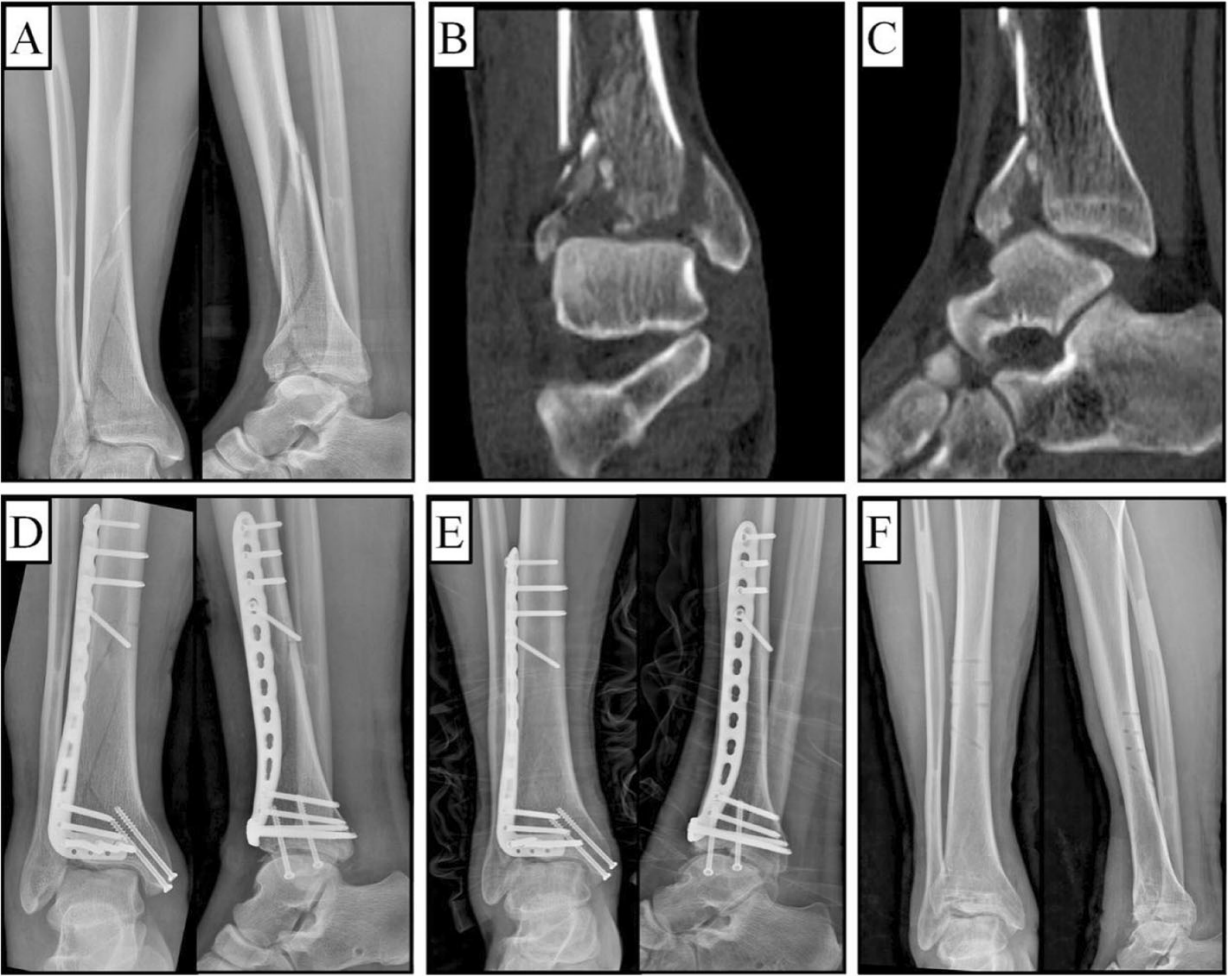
A male patient diagnosed with a 43-C1.1 Pilon fracture. a Preoperative X-rays, b, c coronal and sagittal CT showed spiral fracture of distal tibia and comminuted fracture of anterior distal tibia, and longitudinal fracture of the medial malleolus, as well as slight compression of the talar articular. Postoperative AP and lateral X-ray examinations of the patient at d 1 day, e 1 year postoperatively, and f after implant removal indicating slight arthritis
